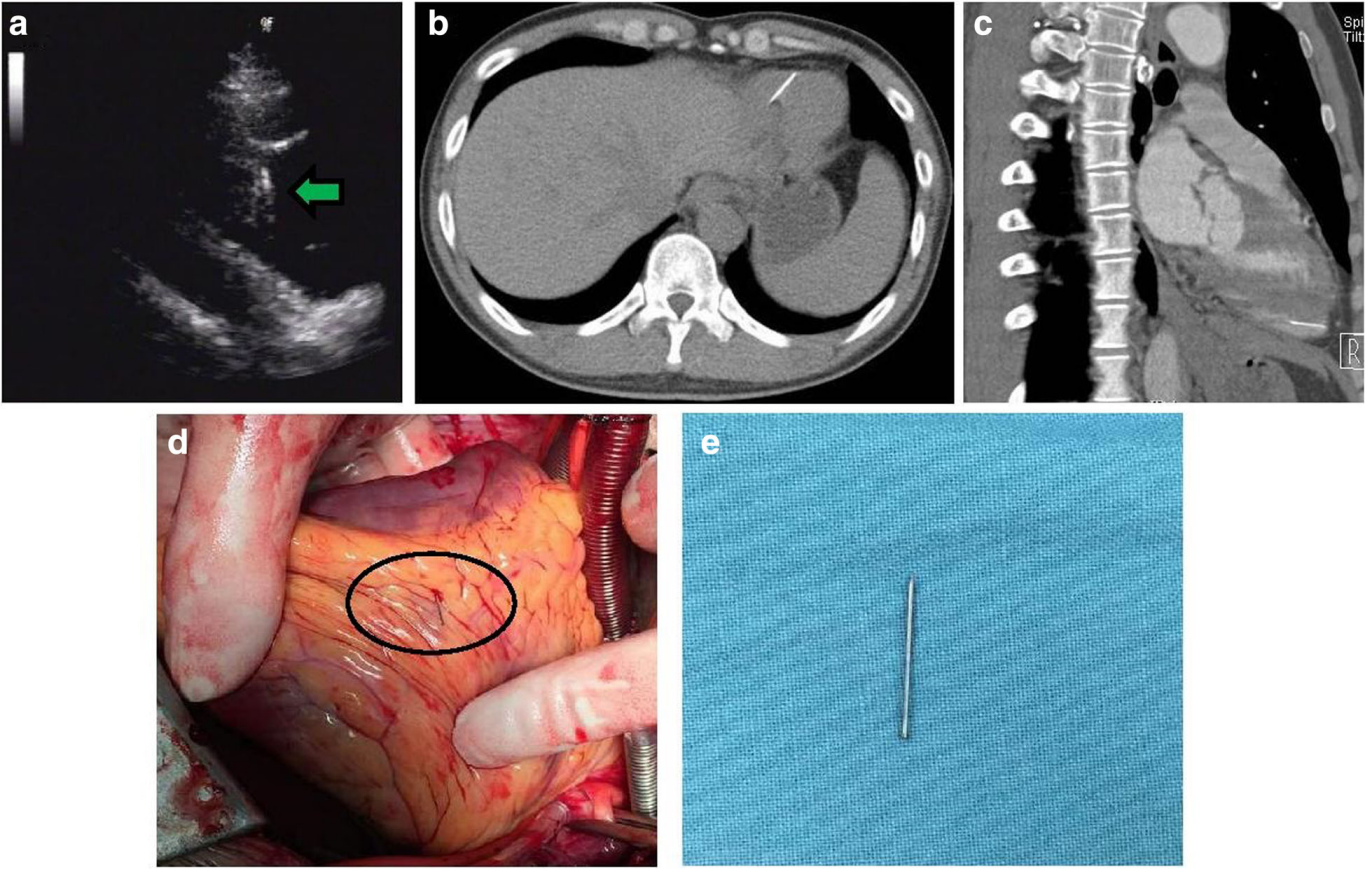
Retained needle fragment in the right ventricle. a Transthoracic echocardiography revealed a retained needle fragment in the inferior wall of the right ventricle, and no detectable pericardial effusion. b and c Computed tomography confirmed the location of a needle in the inferior wall of the right ventricle and near the ventricular septum. d and e The needle was found and removed from the right ventricle
